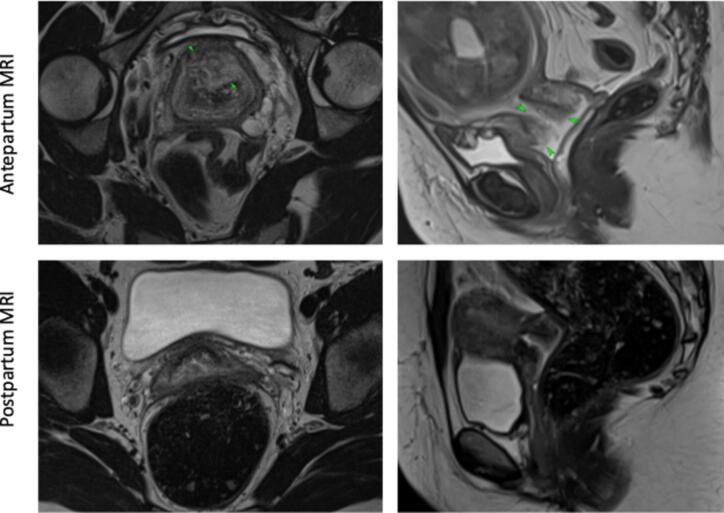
Left panel*, the antenatal magnetic resonance image at 30 weeks gestation shows a large cervical mass in the centre (green arrow). *Right panel,* showing the same area 20 weeks postpartum with normal female genital anatomy and no cervical mass.
